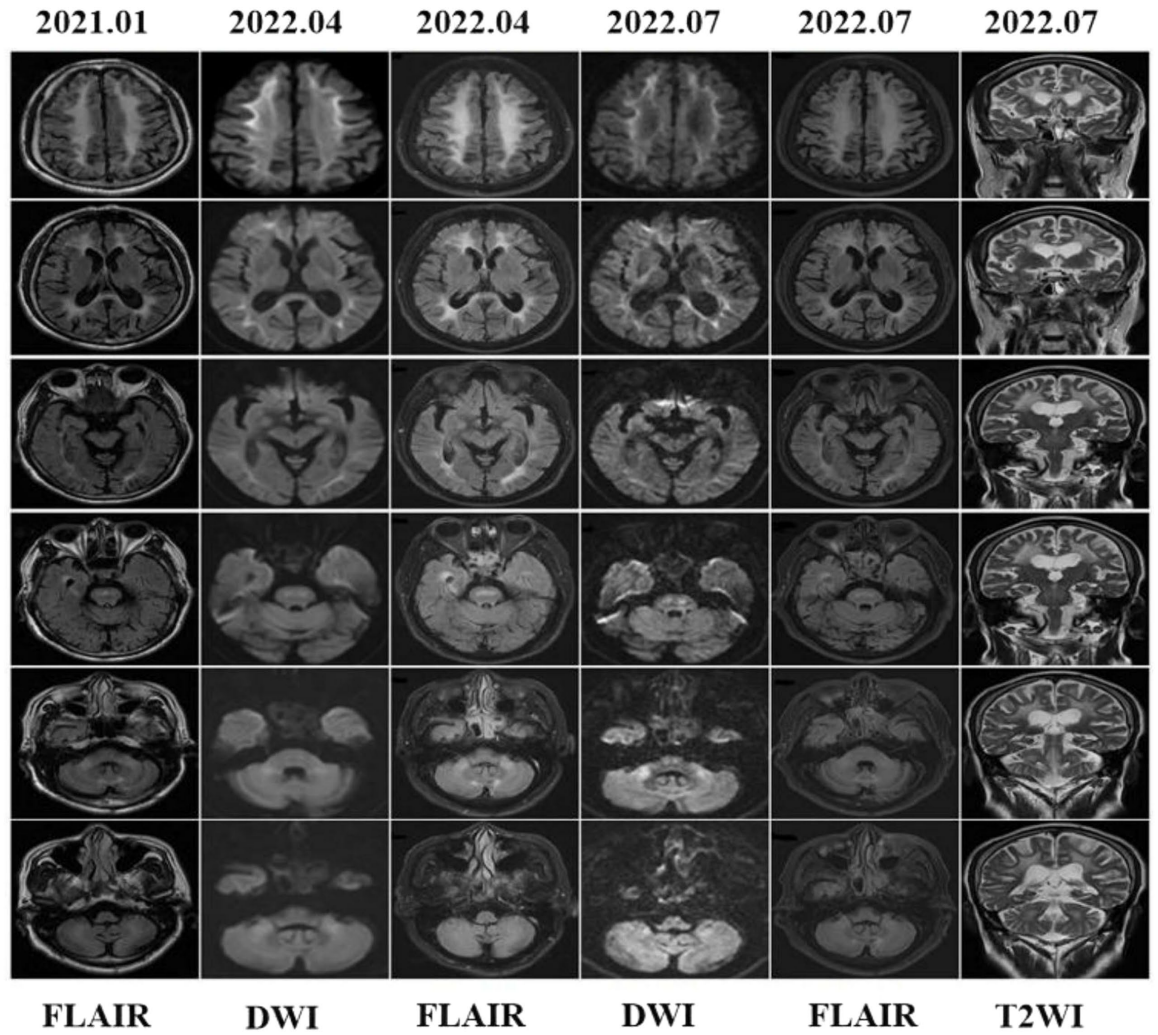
Brain MRI findings showed leucoencephalopathy in fluid attenuation recovery (FLAIR) images, but DWI was not performed in 2021. In 2022, cranial MRI showed high-intensity lesions in the CMJ, the corpus callosum, internal capsules, bilateral cerebral peduncles, middle cerebellar peduncles (MCP sign), paravermal areas and even the cerebellar white matter and brain stem. Brain stem abnormal MRI findings increased over time.
